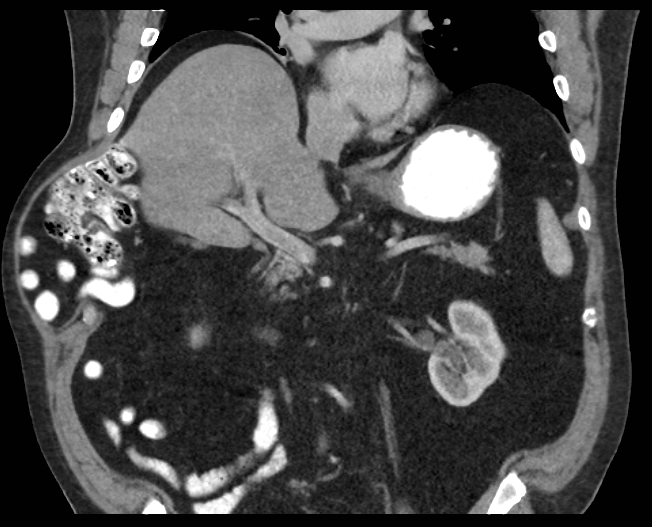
CT Scan of Patient #2 Showing a Similar Defect to Patient #1 in the Right Anterolateral Chest Wall Including Portions of Small Bowel and Portion of Right Colon
